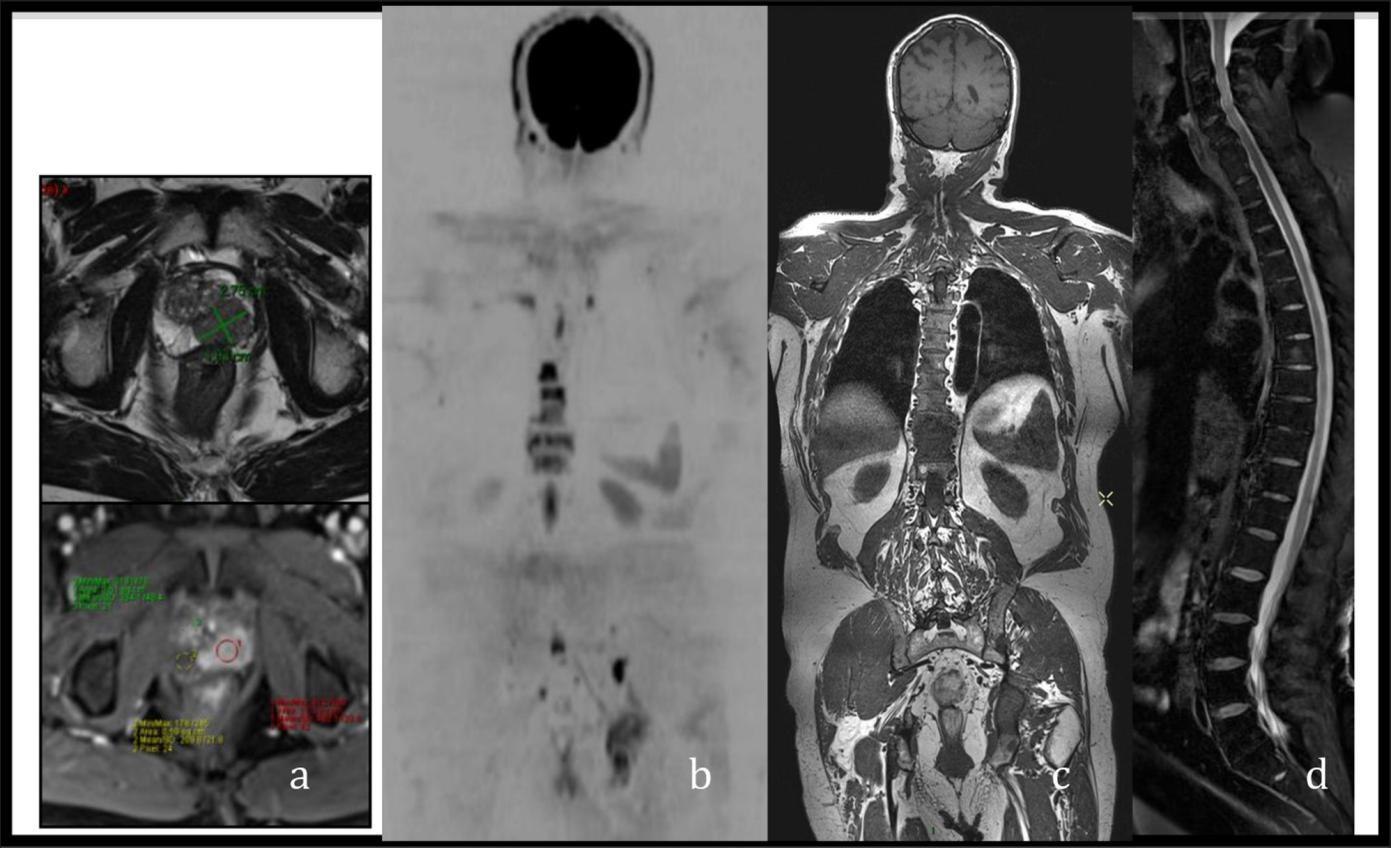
‘All-in-one’ MRI protocol for the concurrent TNM staging of PCa (a) mp-MRI of the prostate for the T staging (b, c) and (d) 3D T1 sequence, DWI and PDFS of the spine for the N and M staging.
